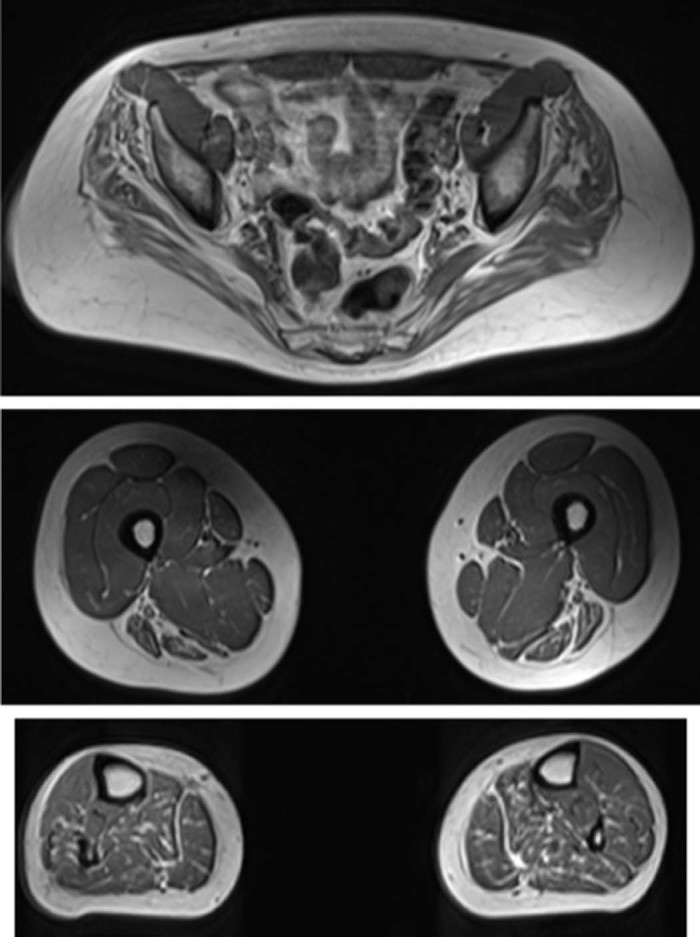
Muscle MRI, T1-weighted axial images, symmetrical atrophy and fat infiltration of the gluteus, hamstrings and calf muscles.
